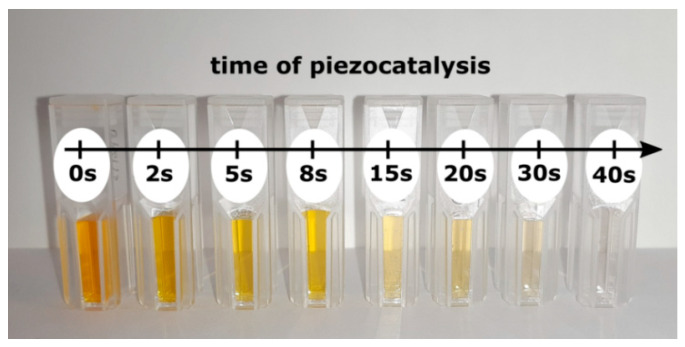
Photograph of the methyl orange (MO) aqueous solutions samples after different times of ultrasonic irradiation. Piezocatalysis was performed using VCX-750 ultrasonic reactor (Sonics & Materials Inc., Newtown, CT, USA) with ultrasound frequency f = 20 kHz and power P = 750 W.
